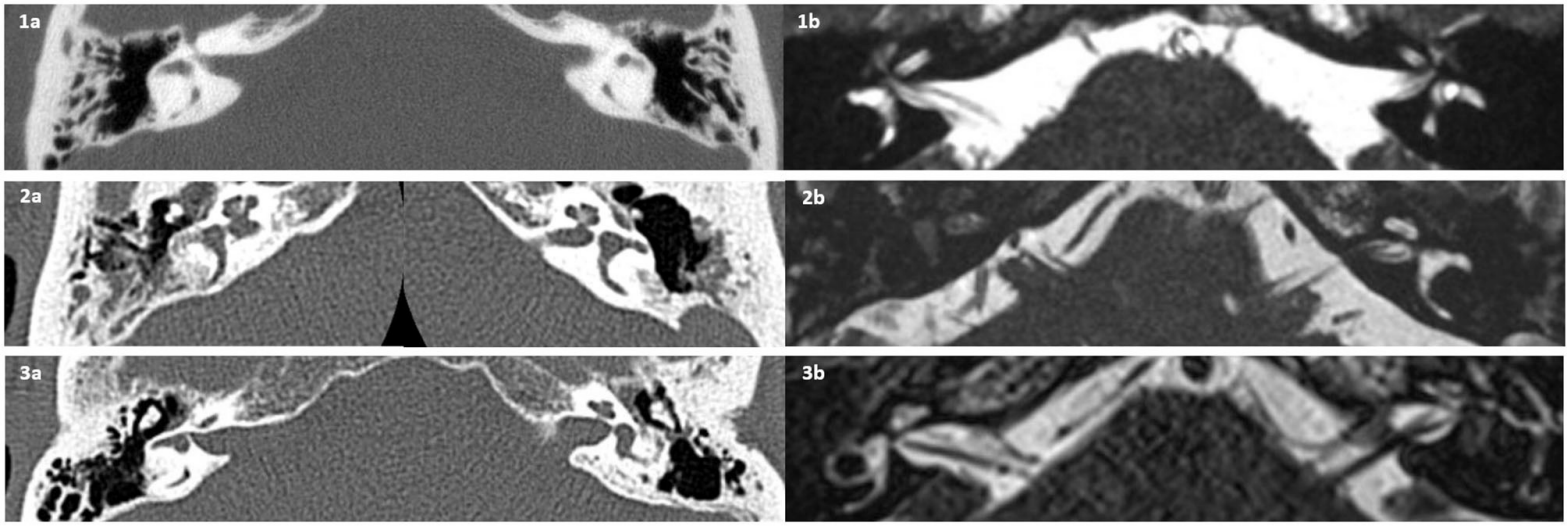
Preoperative temporal bone computed tomography (CT) and magnetic resonance imaging (MRI), axial plane. (1) Pneumococcal meningitis. (1a) CT showing bilateral sclerotic foci in lateral semicircular canal (L-SCC). (1b) MRI showing bilateral hypointense L-SCC fluid signals. The patient demonstrated absent vestibular-evoked myogenic potentials (VEMPs) and complete loss of vestibulo-ocular reflex (VOR) function. (2) Pneumococcal meningitis. (2a) Right-sided L-SCC ossification on CT; left L-SCC normal. (2b) Absent MRI fluid signal of right labyrinth; left side appears normal. The patient had bilateral sensorineural hearing loss, anacusis on right side and PTA 65 left, and bilateral missing cVEMPs. (3) Pneumococcal meningitis and following bilateral cochlear implant (CI). (3a) CT showing normal appearance of the right L-SCC, in contrary to the left L-SCC, which appears irregular, interpreted as ossification. (3b) Decreased left-sided MRI fluid signal and normal on right side. The patient had bilaterally missing cVEMPs, however unilateral VHIT deficit (complete loss of VOR on left side).
